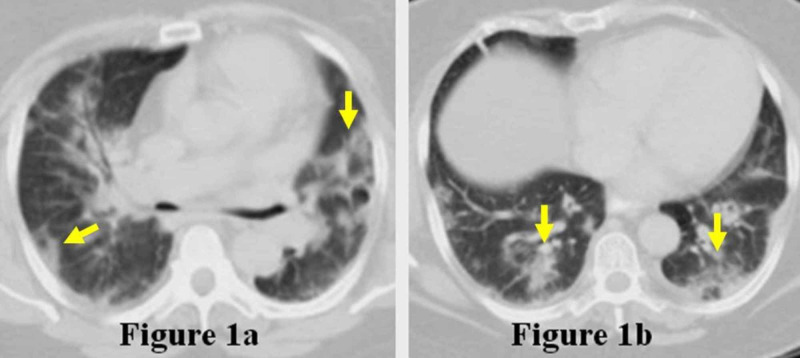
Initial Computed Tomography (CT) of the Abdomen Bibasilar peripheral ground-glass opacities (arrows) in superior (Figure 1a) and inferior (Figure 1b) slices from the initial CT abdomen examination.
